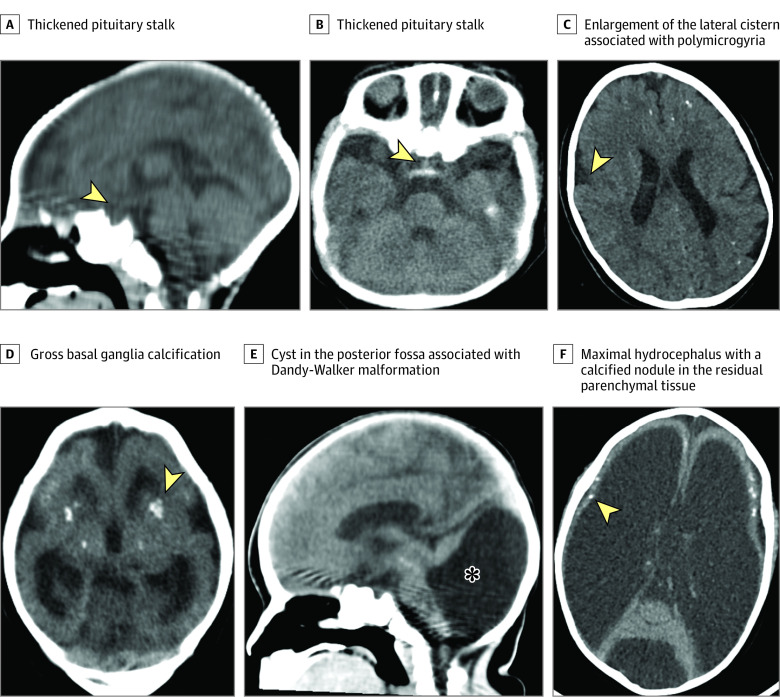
Noncontrast Axial Computed Tomographic (CT) Images and Sagittal Reconstructions of CT Images Panels B, C, D, and F are noncontrast axial CT images. Panels A and E are sagittal reconstructions of CT images. Arrows and asterisk indicate specified features.
